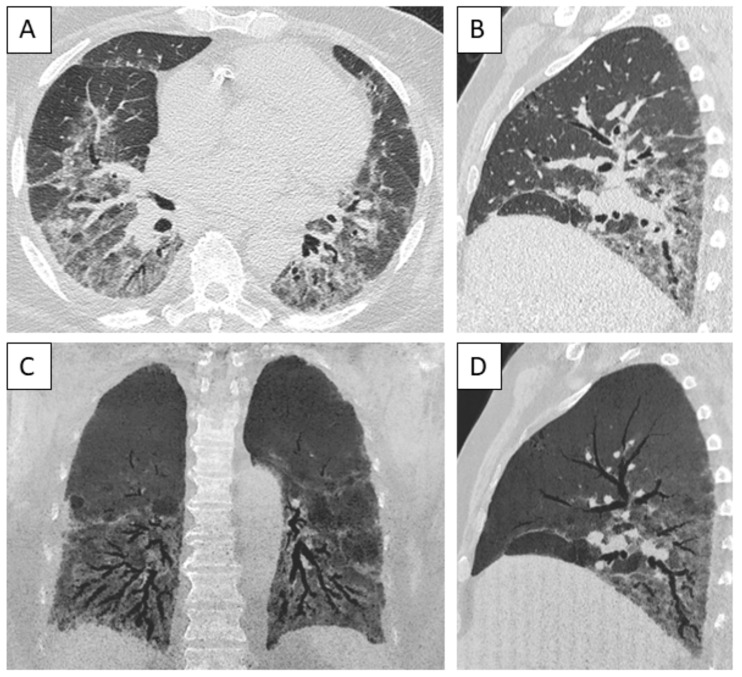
Computed tomography of a male patient with anti-Ku antibodies, demonstrating a pattern of fibrotic nonspecific interstitial pneumonia. (A) Axial view of lower part of the lungs, demonstrating diffuse distribution of ground-glass opacities, reticulation, bronchiectasis, and bronchiolectasis. (B) Sagittal view showing apicobasal distribution. (C) Coronal view, with minimal intensity projection of 13 mm. (D) Sagittal view, with minimal intensity projection of 13 mm.
